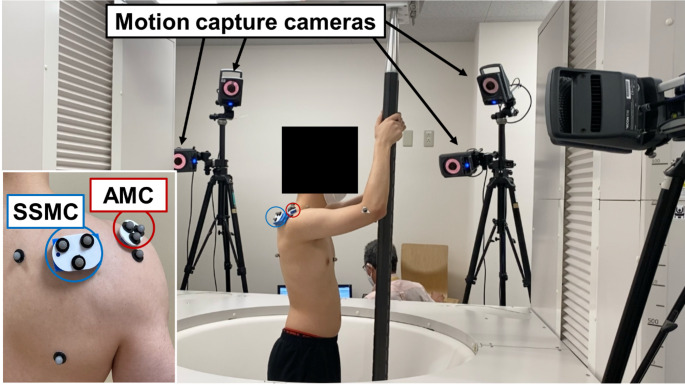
Shoulder elevation tracking using motion capture surrounding the upright CT scanner. An optical motion-capture system is positioned around the upright CT scanner, and motion capture cameras (black arrows) record shoulder motion. The acromion marker cluster (AMC; red circle) and scapular spinal marker cluster (SSMC; blue circle) are used to track scapular movement. Motion-capture data are compared with 4DCT measurements to validate the accuracy of the AMC and SSMC approaches
